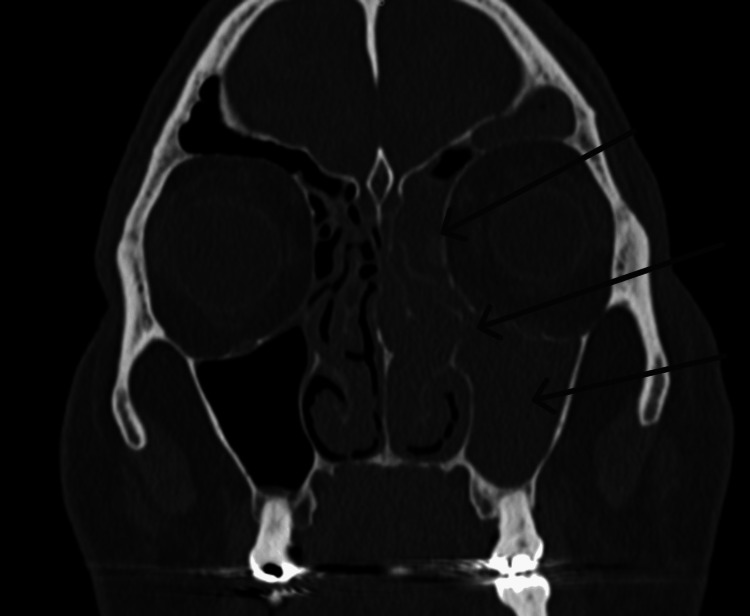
Onset coronal sinus CT scan Evidence of chronic inflammatory changes in the medial wall of the maxillary sinus at the level of the middle turbinate and tissue density in the left frontoethmoidomaxillary region.
